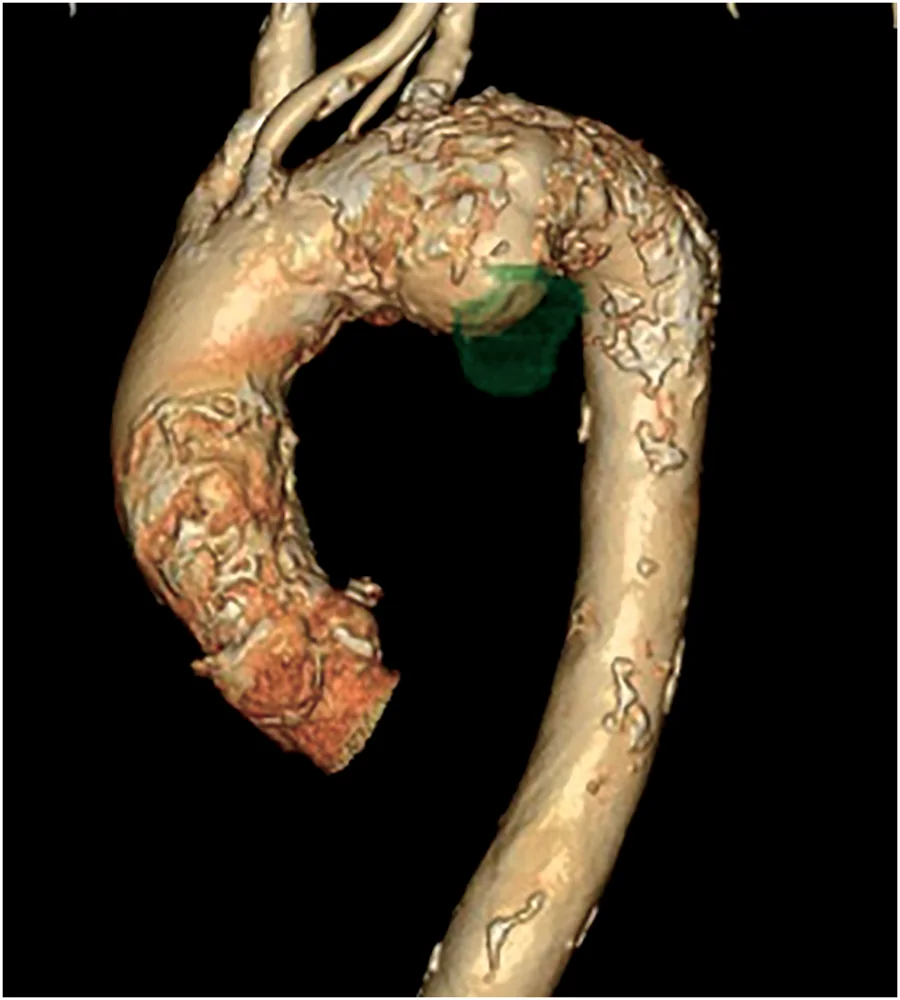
Contrast-enhanced CT shows a 60-mm saccular aneurysm. CT, computed tomography
